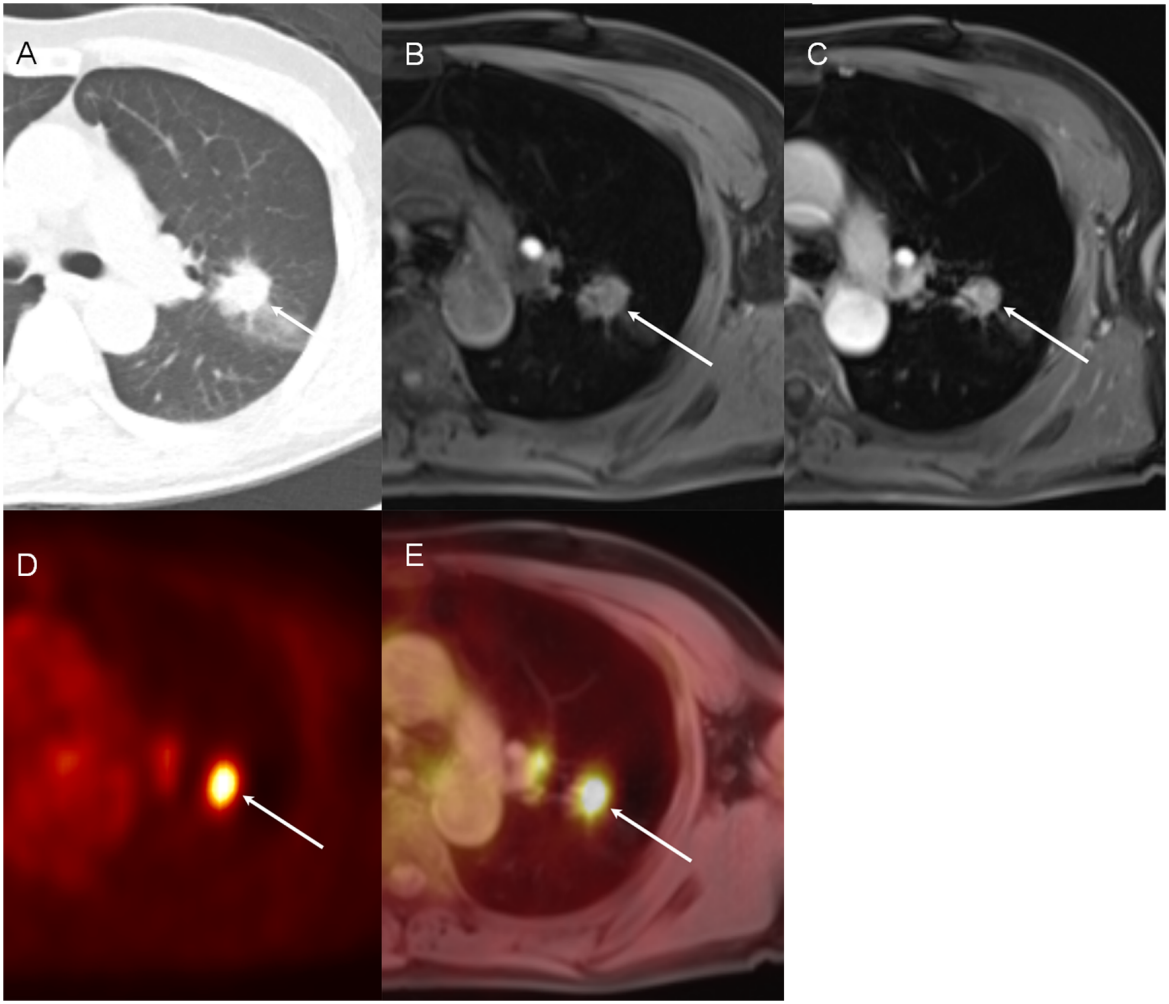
An example of the FDG-avid nodule on PET/MR images (67-year-old man with lung cancer). The primary lung cancer lesion (arrow) is seen on CT image (A), pre-contrast VIBE (B) and post-contrast VIBE (C) images. This nodule (arrow) is also well-delineated as an FDG-avid nodule in the left upper lobe on PET (D) and fused PET/MR images (E).
